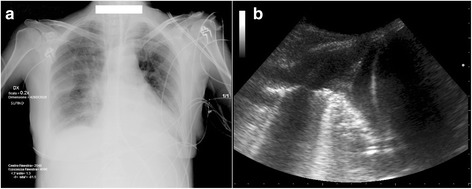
a Supine chest X-ray showing a modest right pleural obstruction. b The corresponding ultrasound image, showing a pleural effusion of about 2 cm
